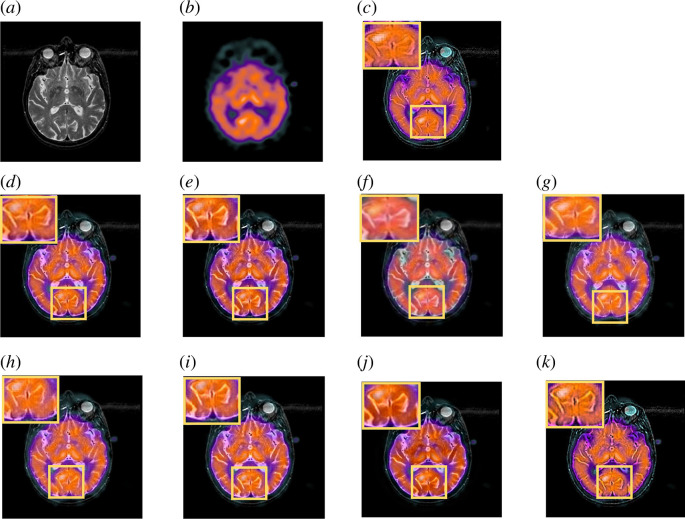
Comparison of fused image on the MRI-SPECT image dataset. (*a*) Original MRI image. (*b*) Original SPECT image. (*c*) MLMG-PCNN [16]. (*d*) JBF [18]. (*e*) Local extreme map GF [39]. (*f*) LRD [7]. (*g*) ACOF [40]. (*h*) MDHU [41]. (*i*) FGF [42]. (*j*) Fusion based on phase congruency [43]. (*k*) Proposed.
